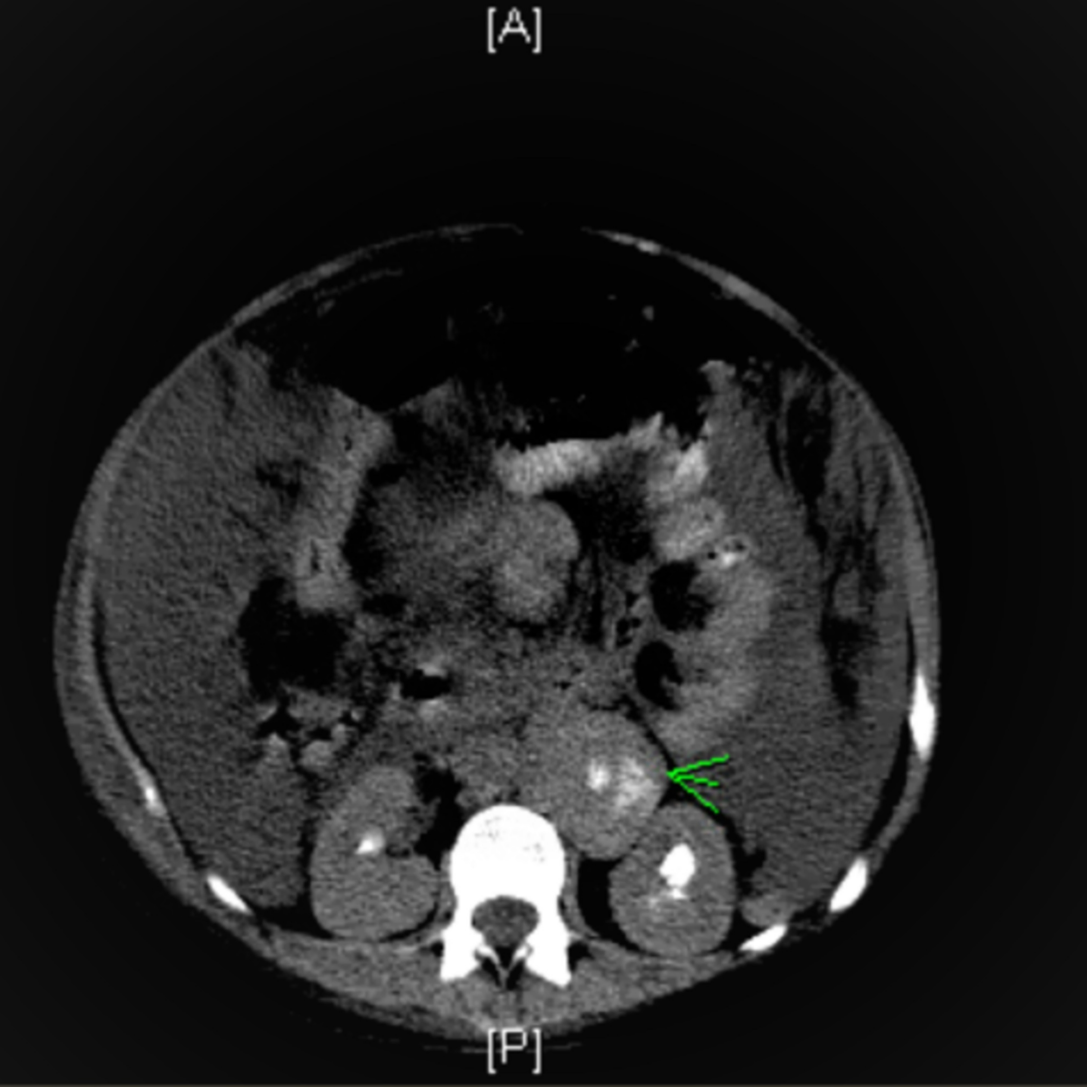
CT scan of the abdomen with the enlarged retroperitoneal lymph nodes containing calcifications
